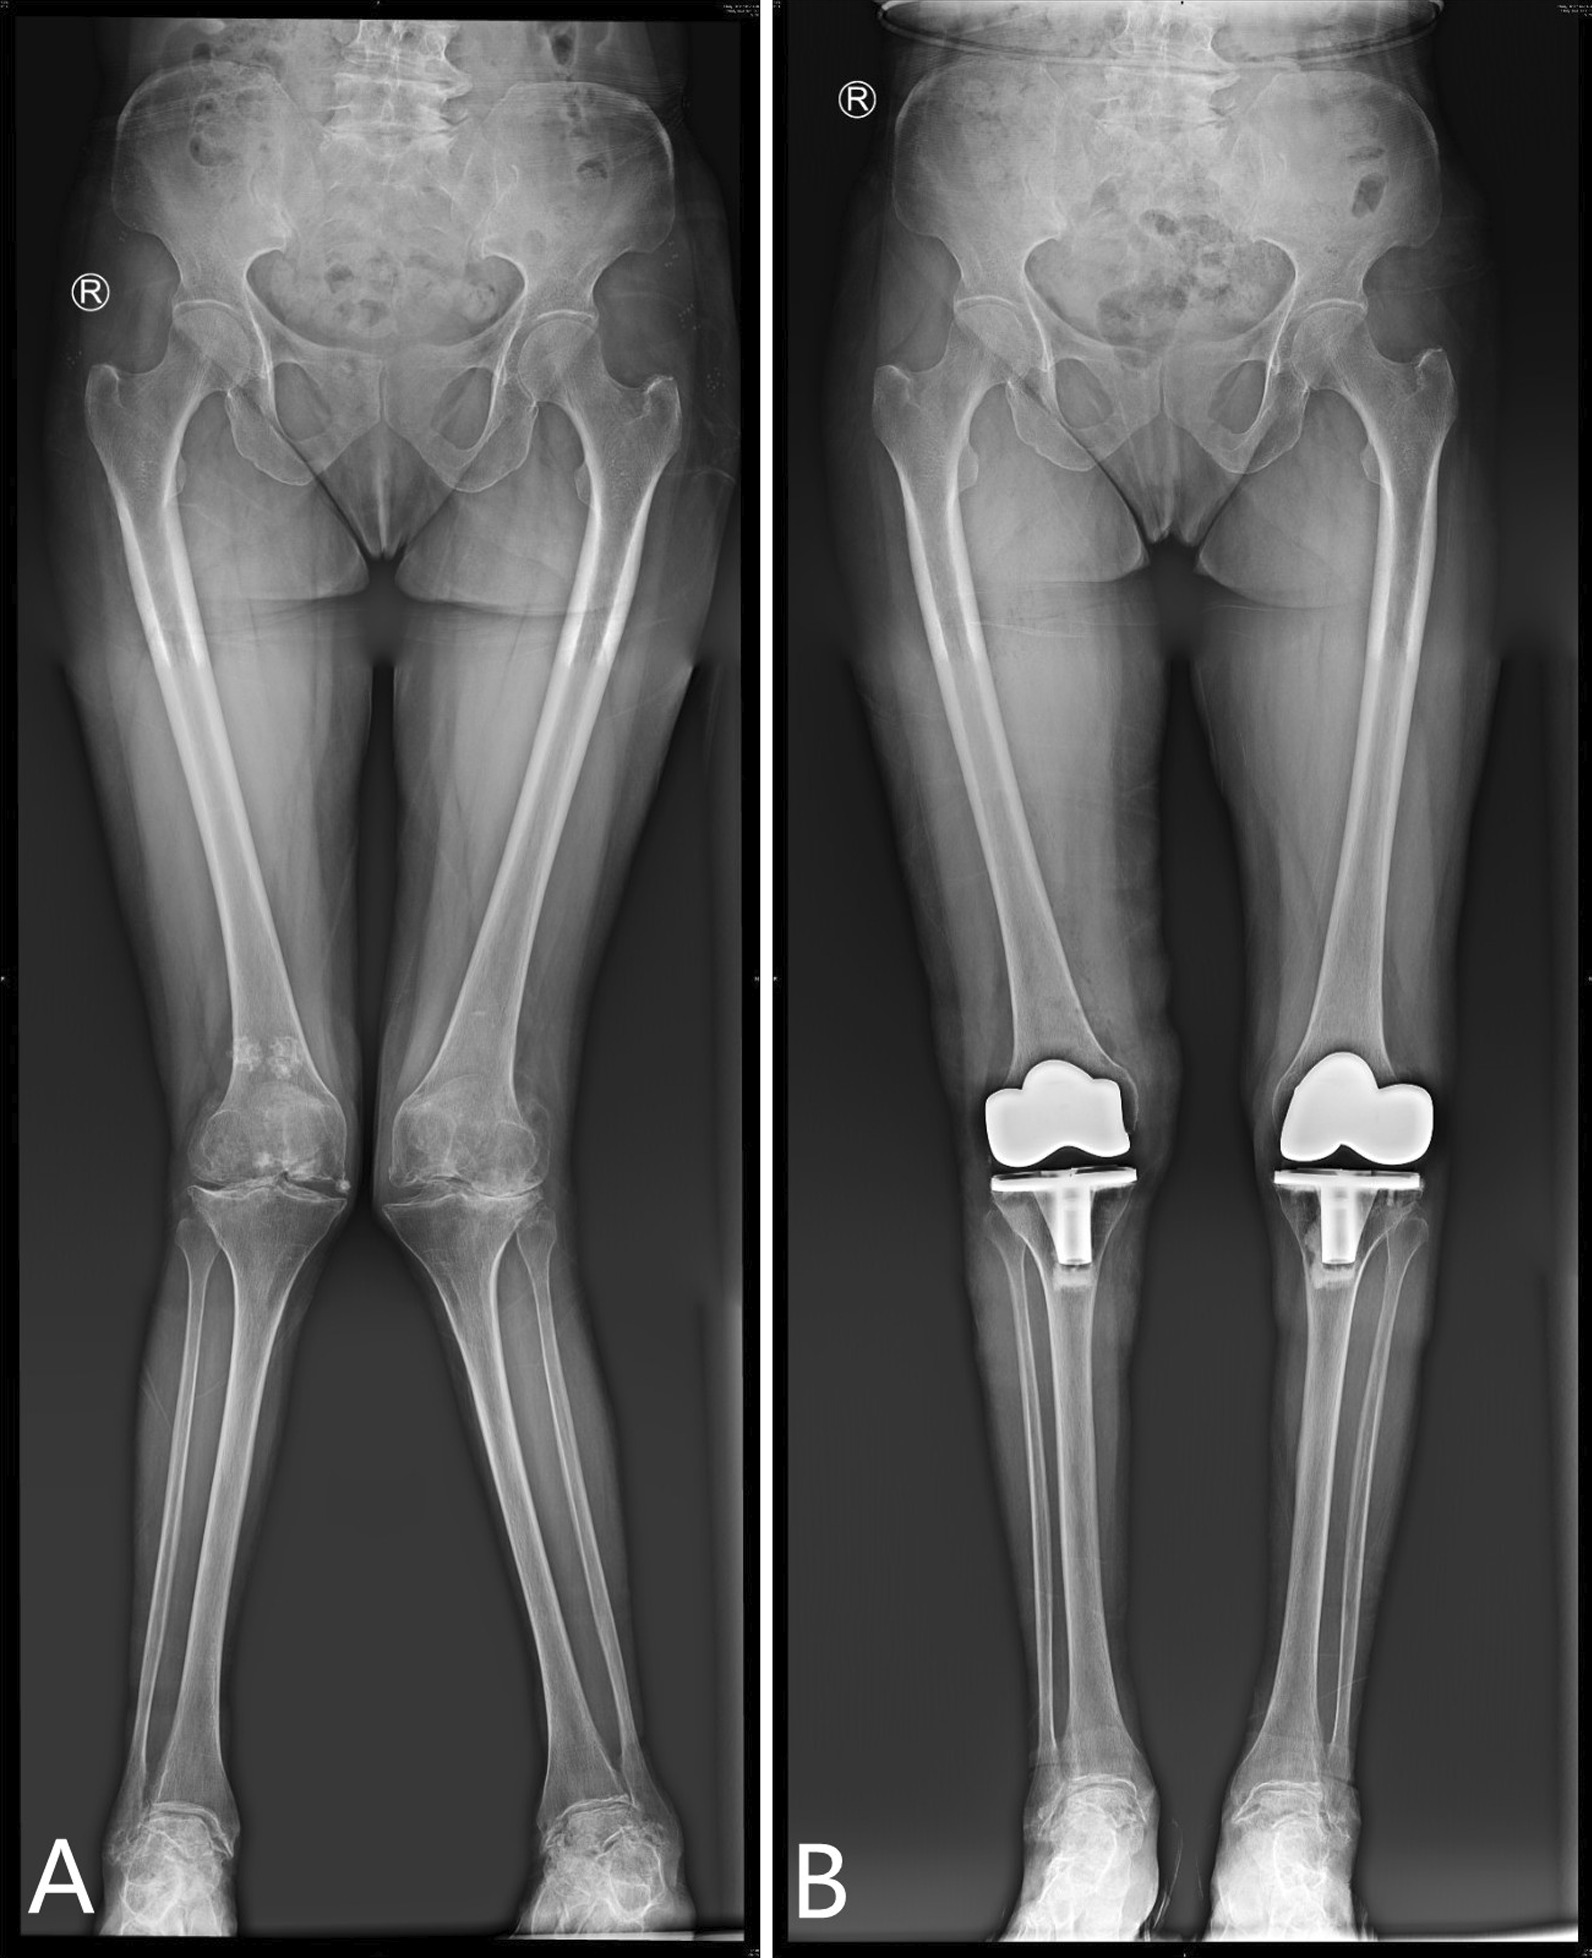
The weight-bearing radiographs of both lower limbs before and after the operation
